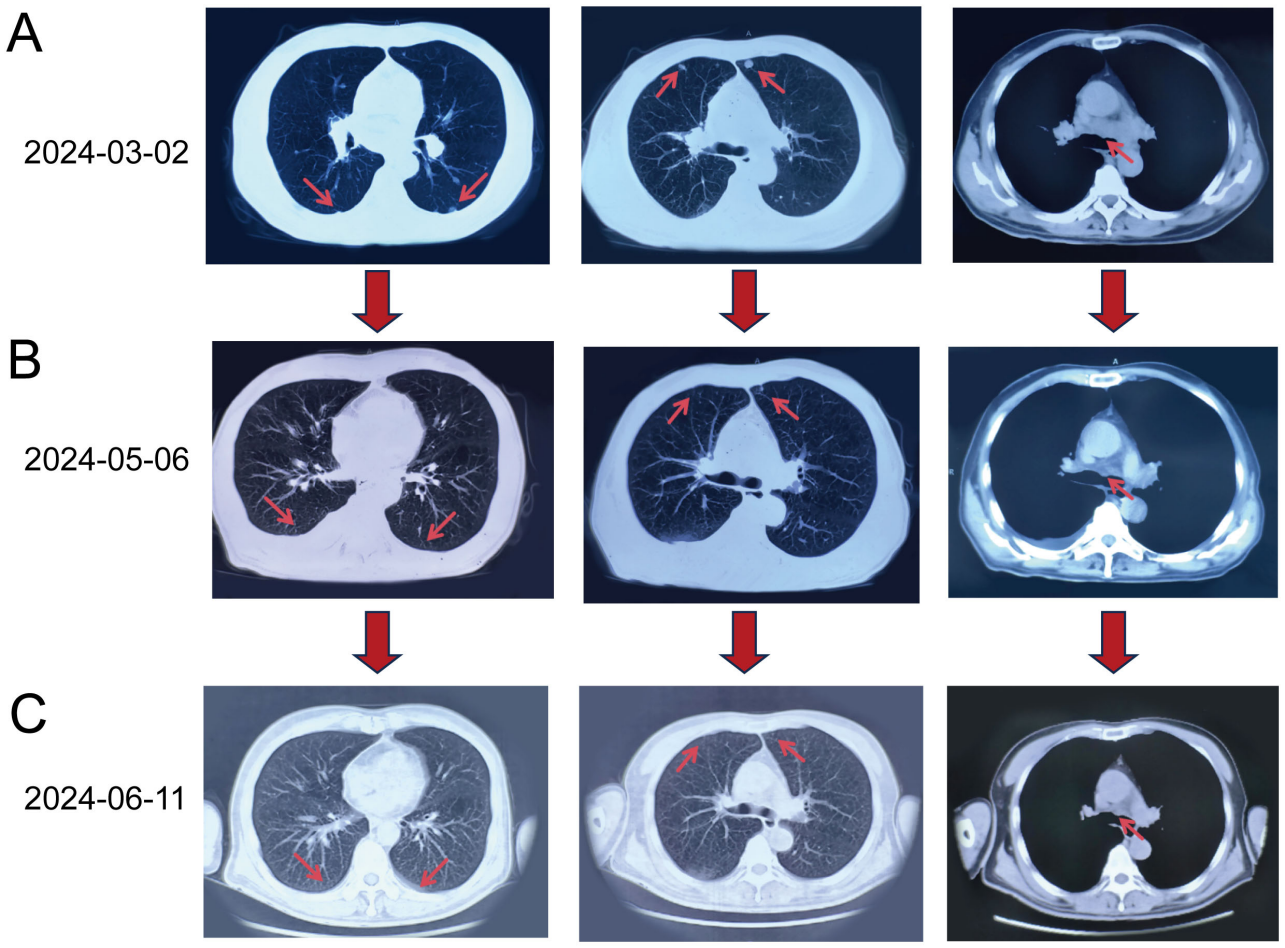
Chest CT scans obtained at different time points. (A) Baseline CT on March 2, 2024: Multiple nodular lesions (red arrows) in both lungs and enlarged mediastinal lymph nodes were visible. (B) Follow-up CT on May 6, 2024 (after four treatment cycles): Reduction in the size and number of pulmonary nodules (red arrows) and mediastinal lymph nodes (red arrow) was evident. (C) CT on June 11, 2024 (after six treatment cycles): Complete resolution of pulmonary nodules (red arrows) and further reduction in mediastinal lymph nodes (red arrow) were observed, indicating a complete response in the lungs.
